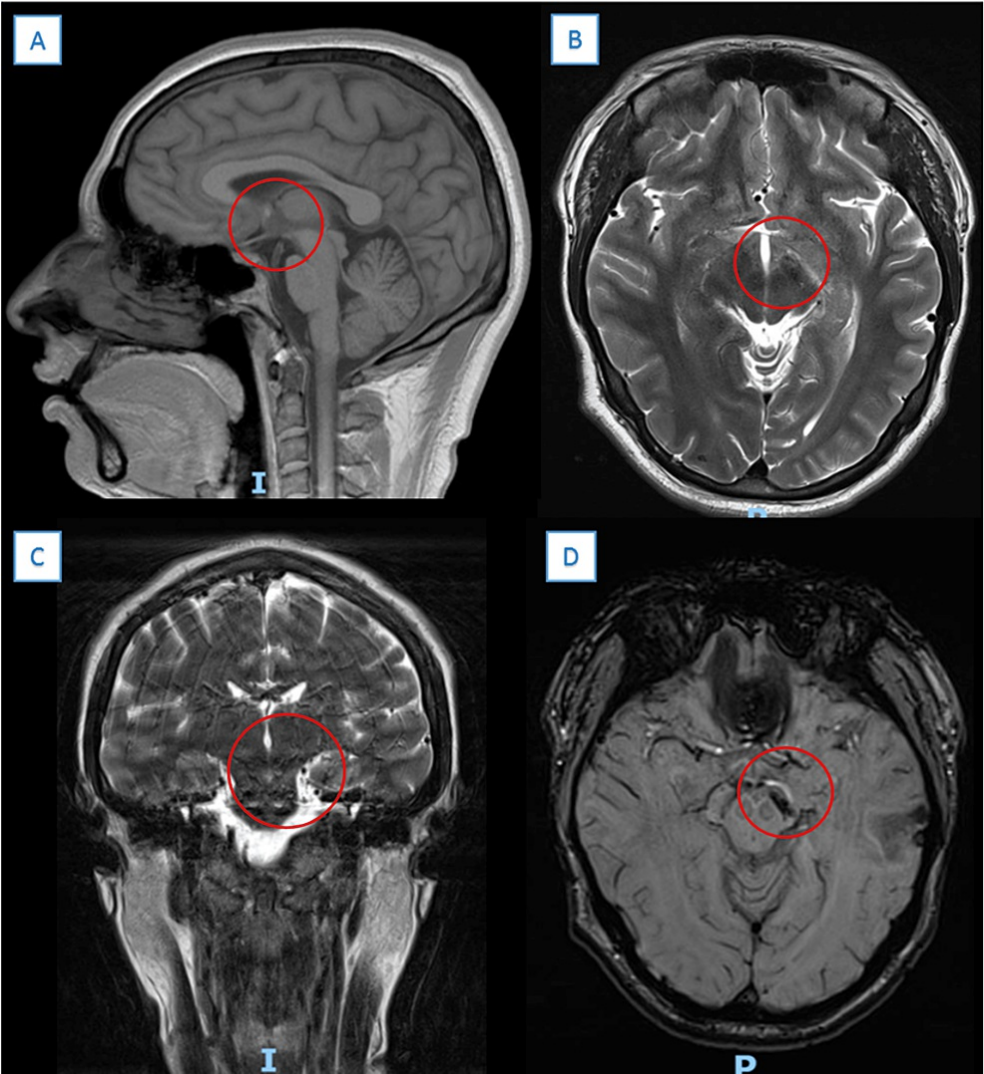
Cranial magnetic resonance imaging (MRI) after one year of surveillance: (A) Sagittal, T1; (B) Axial, T2; (C) Coronal, T2; (D) Axial, susceptibility-weighted imaging (SWI). Encephalomalacia area affecting the most ventral portion of (A) the globus pallidus (red circle), (B, D) the posterior arm of the left internal capsule (red circle), and (C) the left cerebral peduncle. The left cerebral peduncle and ipsilateral pyramid were reduced in size. No new lesions were observed, only chronic post-surgical changes.
